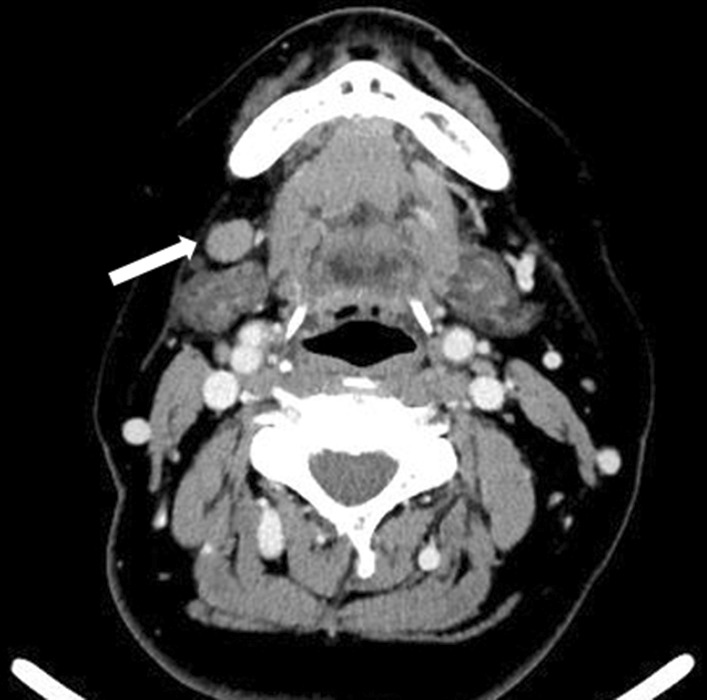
Contrast-enhanced computed tomography showing swelling of the right submandibular lymph node (arrow)
